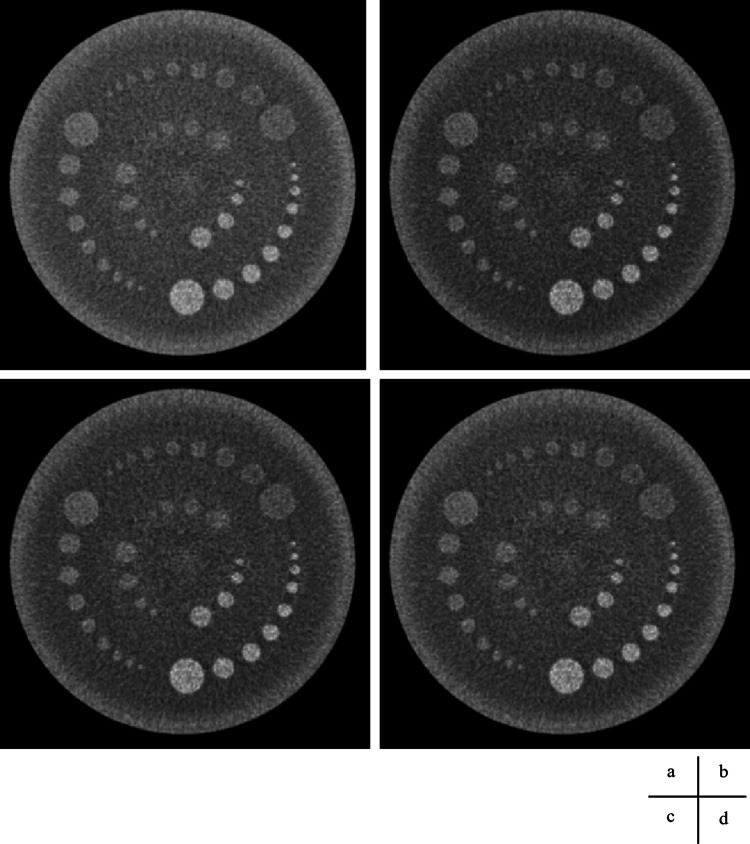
Volume scan and processed images using square transformations, displayed with a wide window. (a) Original CT image: window level (WL) = 50 Hounsfield units (HU); window width (WW) = 40 HU. (b) Square-5 image: WL = 2425 HU; WW = 3600 HU. (c) Square image: WL = 2900 HU; WW = 4000 HU. (d) Square+5 image: WL = 3425 HU; WW = 4400 HU.
